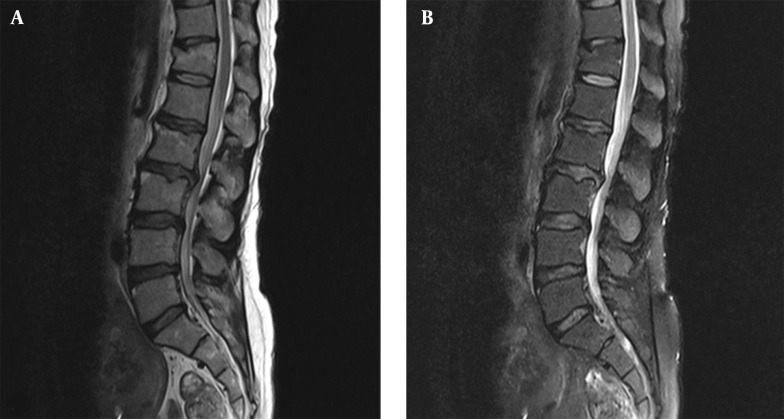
A, T1 weighted; and B, T2 weighted magnetic resonance imaging of the lumbar spine indicating canal stenosis at levels L2-L4
